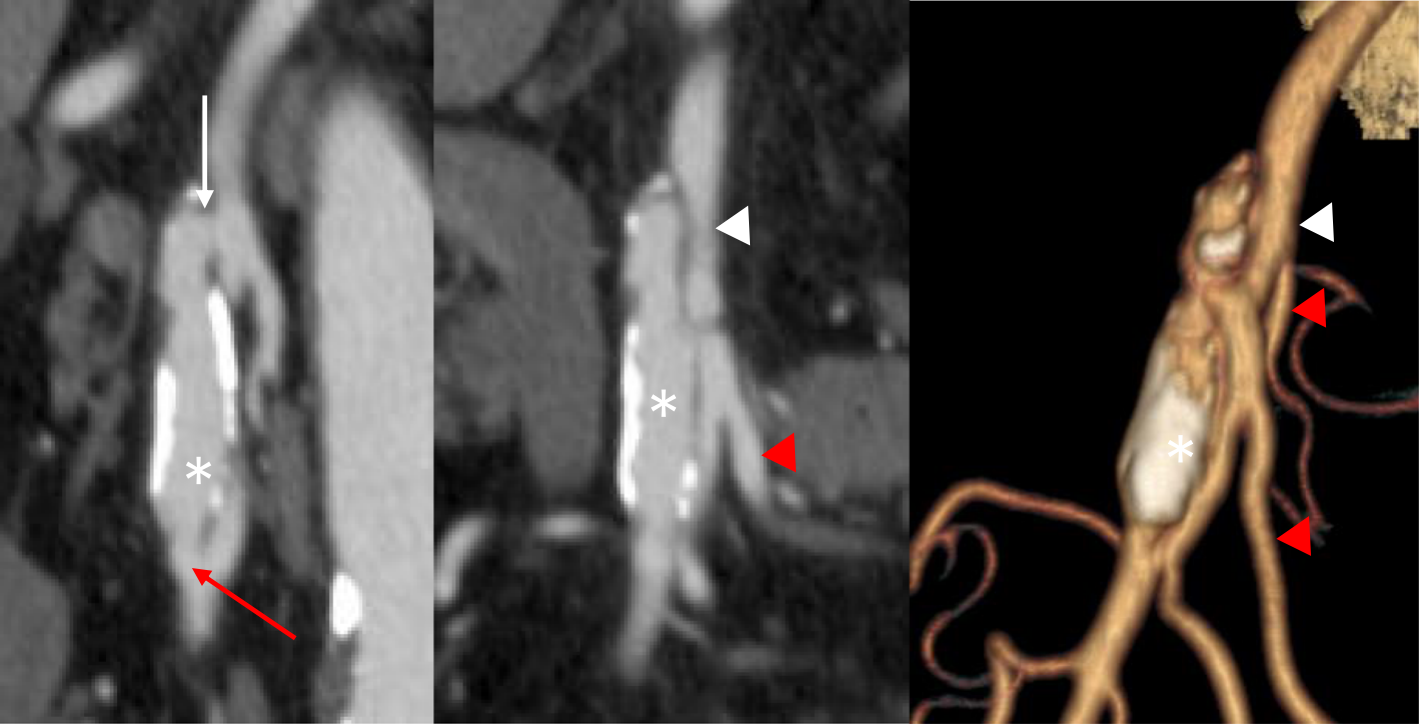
Abdominal contrast-enhanced computed tomography (CT) scans. Multiplanar reconstruction and 3-dimensional CT showed the spontaneous isolated superior mesenteric artery dissection measuring 35 × 6.6 mm in diameter. *: false lumen; white arrow: entry; red arrow: re-entry; white arrowhead: true lumen; red arrowhead: jejunal artery
